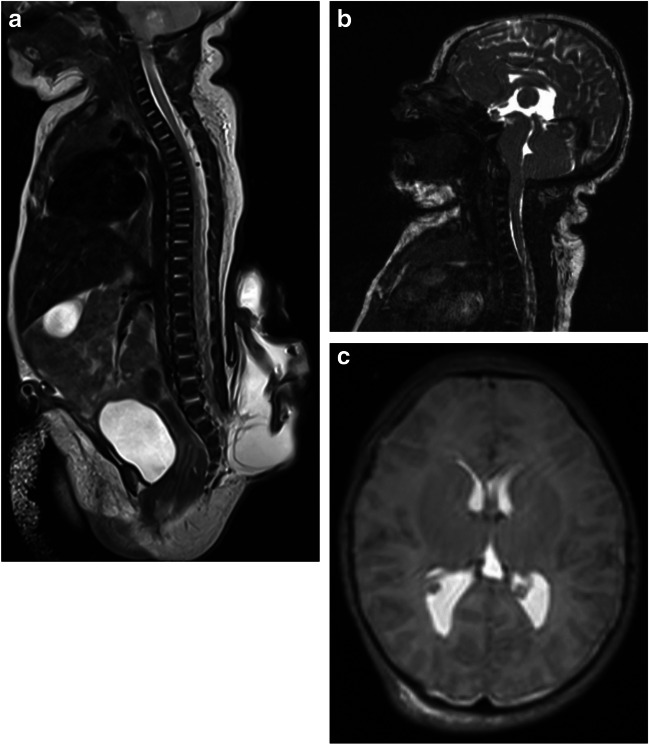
The initial MRI taken before the primary surgical repair of MMC, revealing A a lumbosacral MMC and B intracranially a mild variant of CM2 with reduced volume of posterior cranial fossa, beaked tectum, downward herniation of the vermis, and reduced cerebrospinal fluid (CSF) space in the foramen magnum, C while supratentorial ventricles were small
